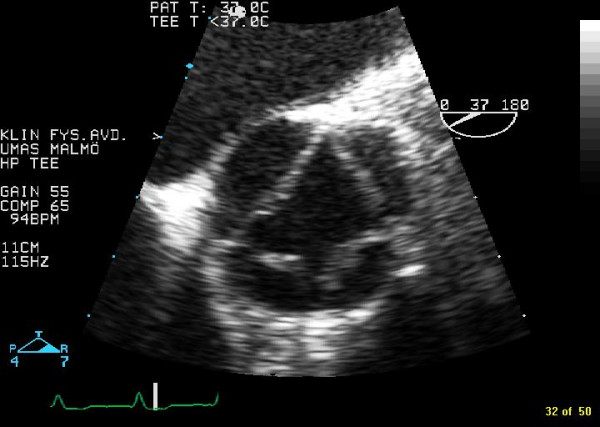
Transoesophageal images of a quadricuspid aortic valve in systole.
